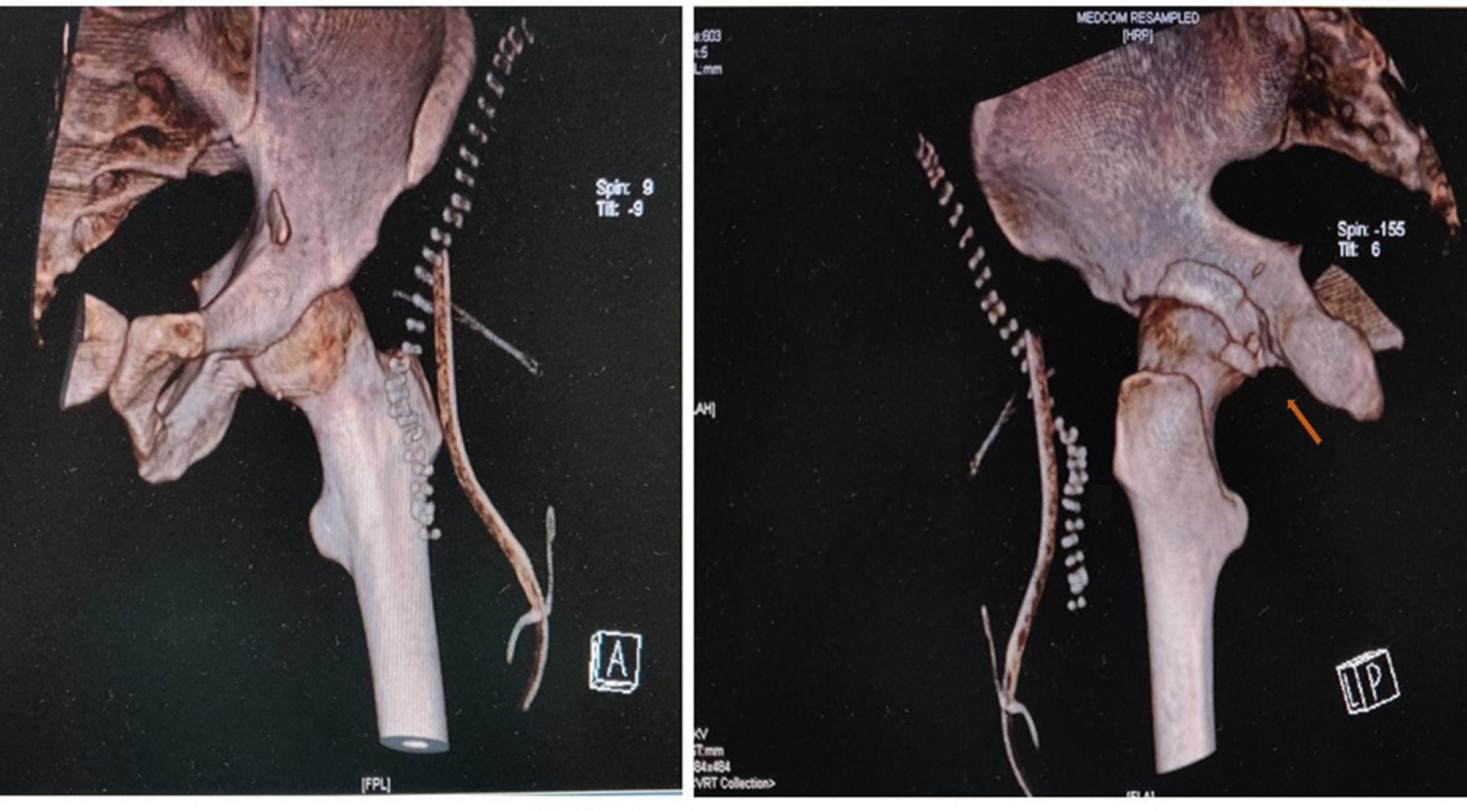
Post-reduction computed tomography (CT) Three-dimensional (3D) cut section of the left hip with pelvis showing the complete reduction of the posterior wall fragment following open reduction
